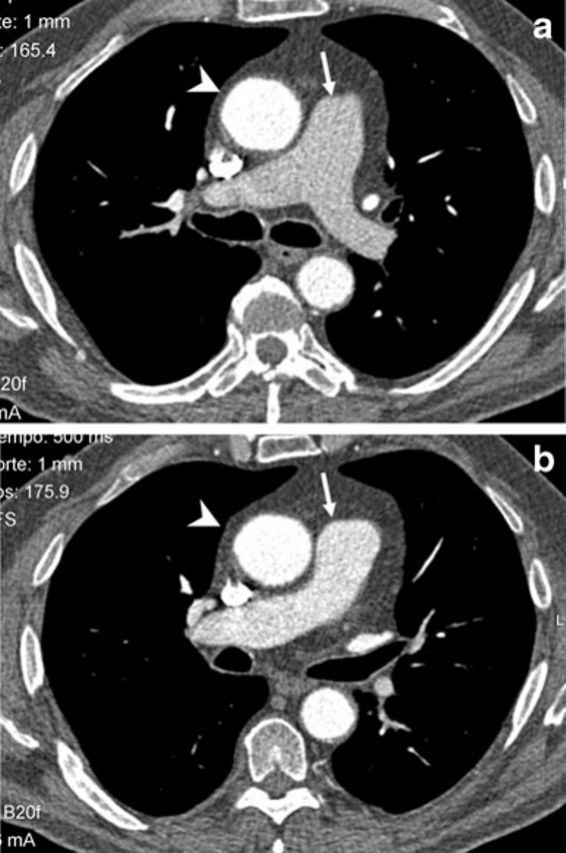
Transverse CT pulmonary angiogram at the level of the pulmonary trunk bifurcation (a) and 1 cm below (b) in a 59-year-old man. Pulmonary artery attenuation (*arrows*) is around 220 HU in a and shows higher density in b (around 300 HU), but the pulmonary artery is less enhanced than the aorta (*arrowhead*), pulmonary veins, and superior vena cava. Patient was considered as presenting a grade 3 transient interruption of contrast (TIC) by both observers
